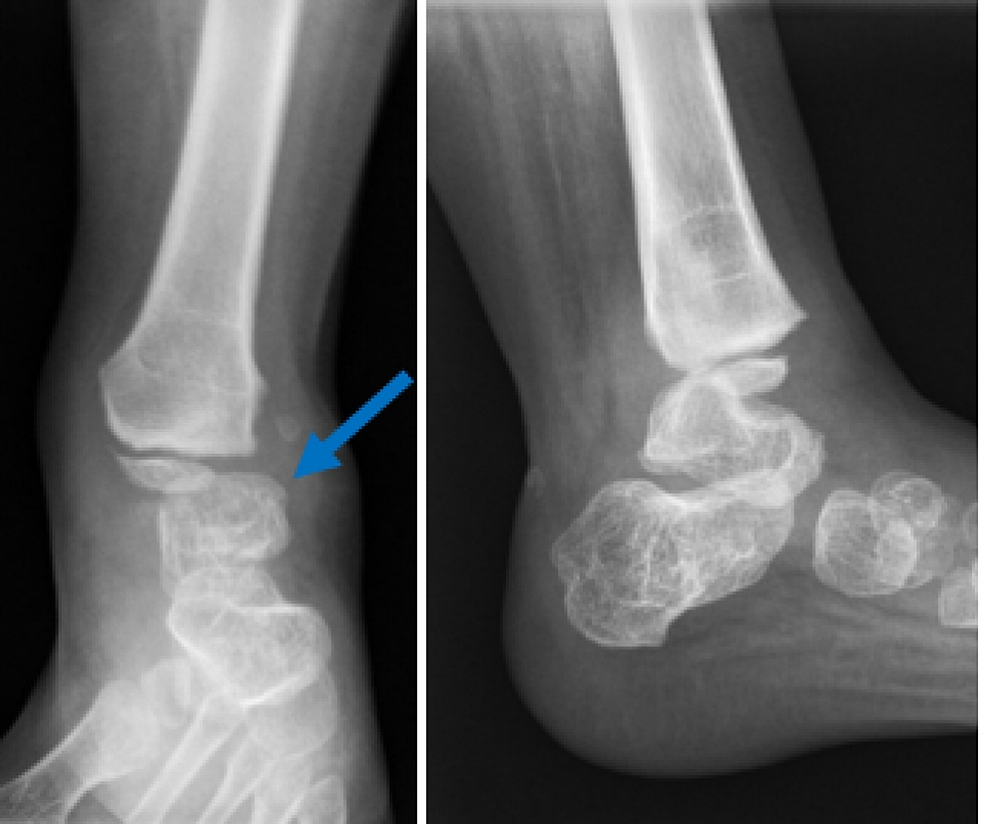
Radiography with deformity Anteroposterior (Panel A) and lateral (Panel B) views of the left ankle 21 months after fibular resection demonstrate superolateral subluxation of the talus with valgus deformity of the ankle.
